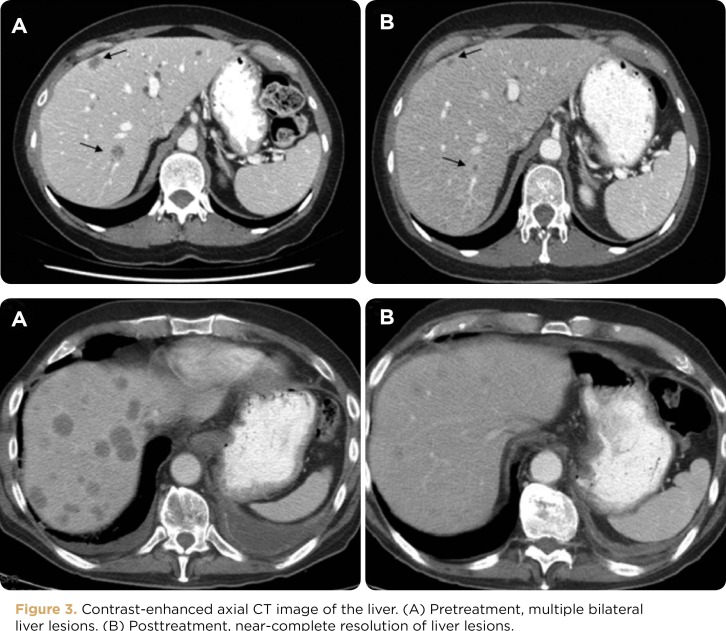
Figure 3. Contrast-enhanced axial CT image of the liver. (A) Pretreatment, multiple bilateral liver lesions. (B) Posttreatment, near-complete resolution of liver lesions.
